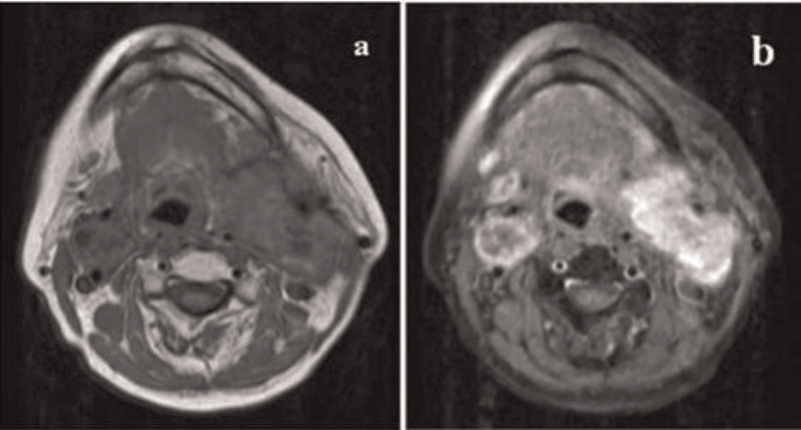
Axial T1-weighted magnetic resonance imaging (MRI) scans. (a) A 5 cm hypointense and isointense with muscle mass on the left carotid bifurcation compressing jugulary vein and carotid artery and 2.5 cm mass at the left side. (b) These masses intensely enhance contrast.
